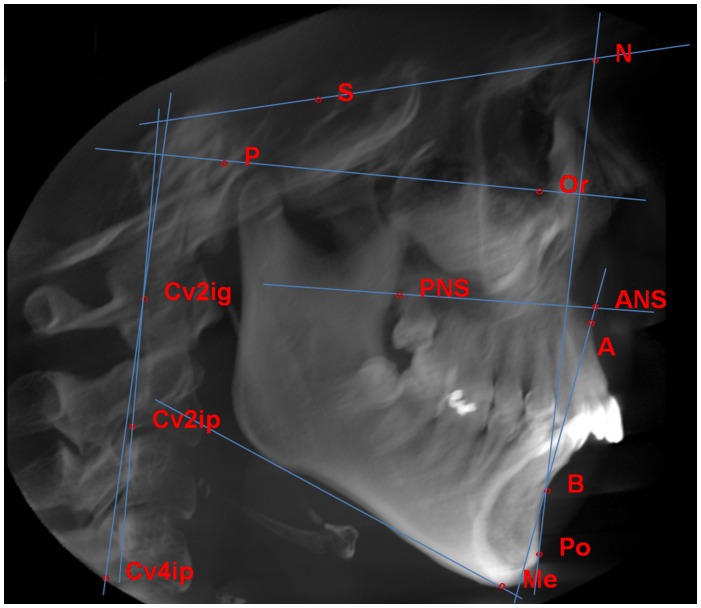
Landmarks involved in the vertical skeletal and head posture measurements on cephalograms from CBCT.
